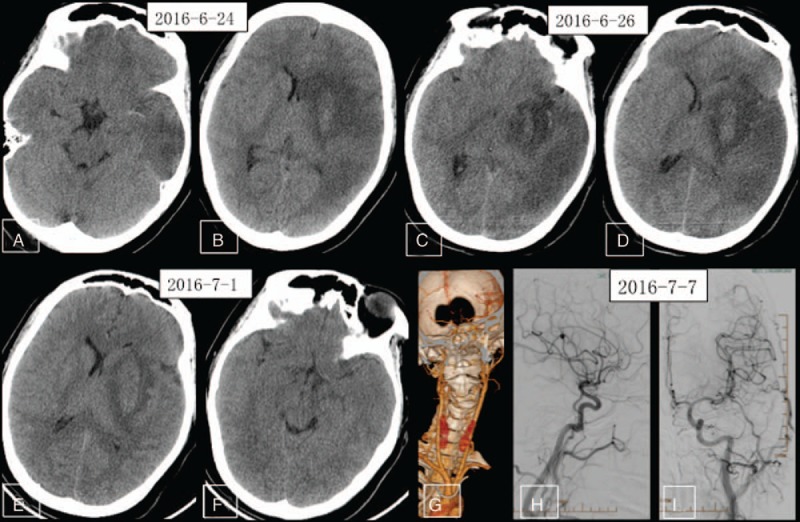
Follow-up cranial CT, CT angiography (CTA), and digital subtraction angiography (DSA). (A, F) Repeated cranial CT showing large infarction lesion with brain edema and HT; (G) repeated CT angiography showing spontaneous recanalization of the occluded left MCA complicated by MCA dilatation (but normal cervical artery structure). (H, I) Digital subtraction angiography (DSA) showing spontaneous recanalization of the left MCA with artery dilatation. CT = computed tomography, CTA = CT angiography, DSA = digital subtraction angiography, DWI = diffusion-weighted imaging, HT = hemorrhagic transformation, MCA = middle cerebral artery.
